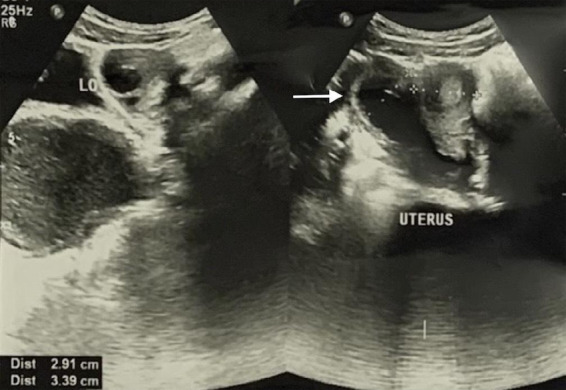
Ultrasound showing uterine didelphys.
